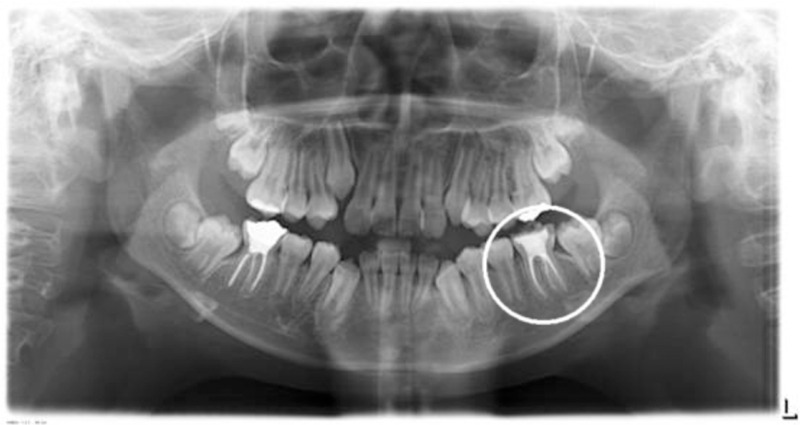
Radiographic appearance after 6 months showed the lesion disappeared.
